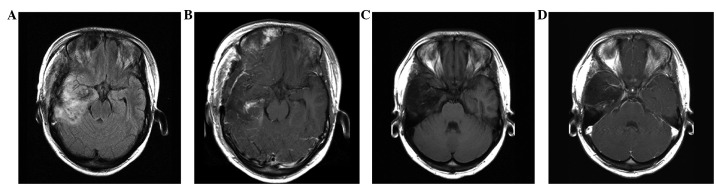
MRI following the initial surgery at (A and B) three days post-surgery and (C and D) one year post-surgery. (A) Slight brain edema in the surgical region (FLAIR sequence imaging). (B) Funicular enhancement, which may have been due to slight hemorrhaging of the brain (contrast-enhanced imaging). (C) T1WI with recurrent manifestation. (D) Contrast-enhanced imaging without any obvious enhancement. MRI, magnetic resonance imaging; WI, weighted imaging; FLAIR, fluid-attenuated inversion recovery.
